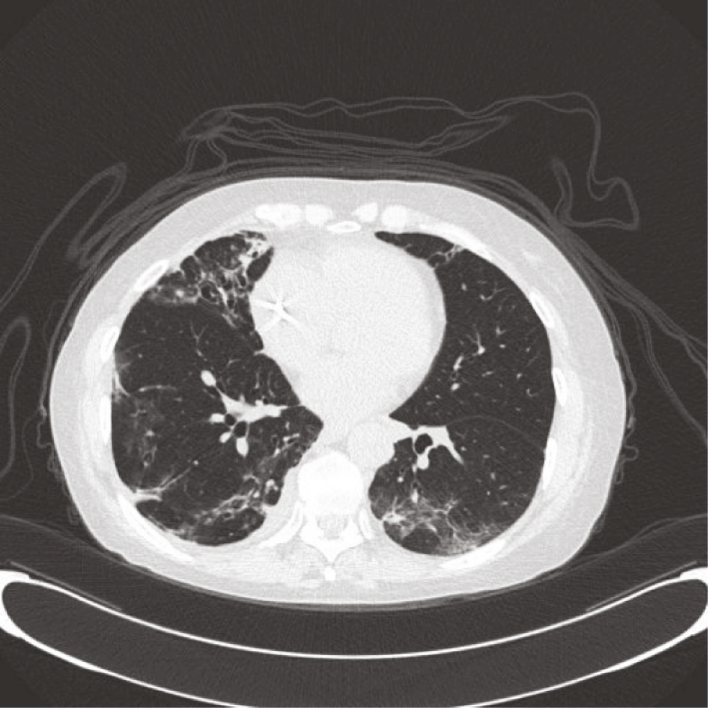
Chest computed tomography example of a case with immune-checkpoint inhibitor induced pneumonitis showing patchy bilateral areas of consolidation and ground-glass attenuation that appeared following initiation of ICI.
